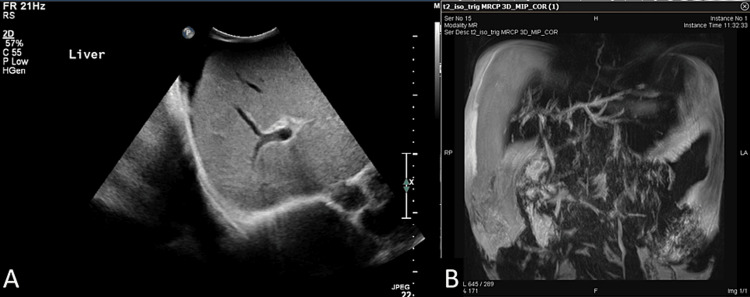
Ultrasound of the abdomen and pelvis and magnetic resonance cholangiopancreatography (MRCP) of the reported patient Figure 1A: An ultrasound of the abdomen and pelvis reveals an enlarged liver measuring 18 cm and homogeneous parenchyma with no focal lesions or intrahepatic biliary dilatation. The portal vein and common bile duct are unremarkable. The gallbladder is surgically removed. The pancreas and both kidneys are unremarkable. The spleen is enlarged, measuring 18 cm, with no focal lesions. There is no ascites. Figure 1B: The MRCP shows two focal areas of cholangitis in the right liver lobe associated with a beaded appearance, suggestive of sclerosing cholangitis with multiple unchanged liver lesions, which represents mostly hepatic abscesses; however, cystic neoplasm could not be totally excluded. There are no radiological signs of pancreatitis.
